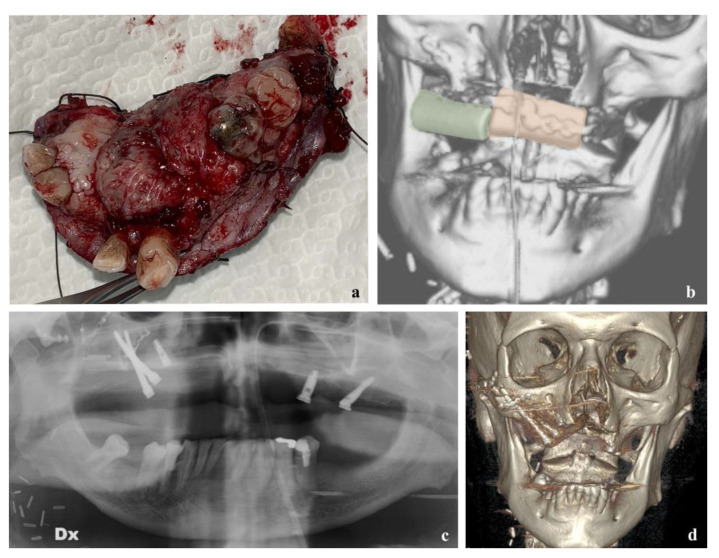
Total flap failure in a patient operated to remove an oral SCC of the maxilla. The patient was affected by epidermolysis bullosa, an autoimmune disease which might have co-played a role in flap failure. (a) Right hemi-maxillectomy, including the carcinoma. (b) Three-dimensional view of the reconstruction achieved by a two-pieces fibula flap. (c) Post-operative OPG showing absence of the necrotic segments. Total flap failure occurred on POD 13. The removal of the necrotic flap was followed by positioning of two right zygomatic implants, three traditional endosseous implants and a temporalis flap. (d) Three-dimensional post-operative CT showing the correct positioning of the implants and the osseous defect.
